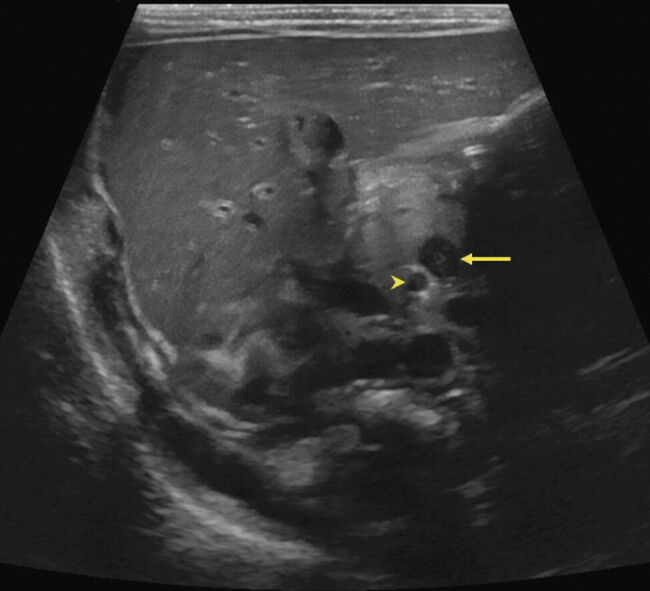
Abdominal ultrasound. Focused sonographic imaging of the mid abdomen shows reversal of the normal SMA to SMV relationship, indicating intestinal malrotation. The SMV (arrow) is located to the left of the SMA (arrowhead), which is a reversal of the normal SMA/SMV relationship. Real-time sweep of the mesenteric axis showed swirling of the mesentery around the SMA (not shown). SMA, superior mesenteric artery; SMV, superior mesenteric vein.
